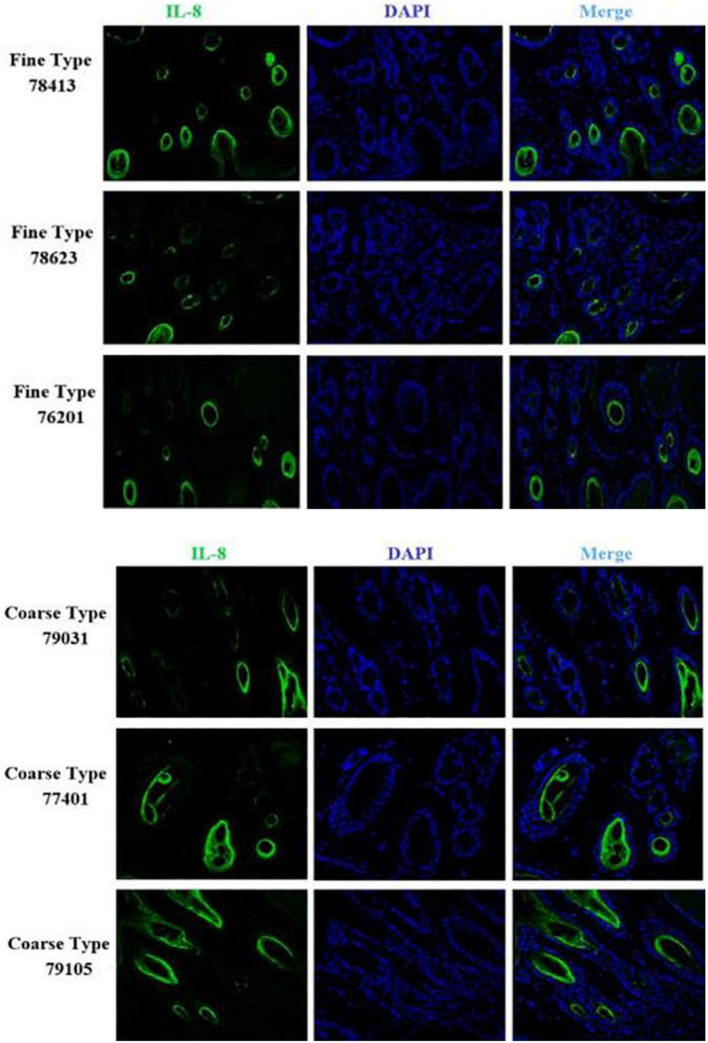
IF results of *CXCL8* in FT-LCG and CT-LCG skin tissues (200x).
